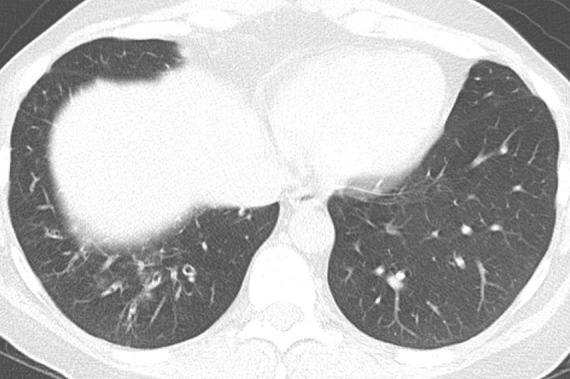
Comparison of traction bronchiectasis with bronchiectasis from chronic airway inflammation. (a) Axial CT scan demonstrates traction bronchiectasis in a patient with fibrotic nonspecific interstitial pneumonia. Note the substantial reticular abnormality and architectural distortion adjacent to the irregular dilated airways. (b) Axial CT scan demonstrates cylindrical bronchiectasis in the right lower lobe of a patient with underlying immunodeficiency. In contrast to traction bronchiectasis, this postinflammatory bronchiectasis lacks adjacent fibrosis and “tethering” of the airways. There are also associated findings of bronchial wall thickening and mucoid impaction.
